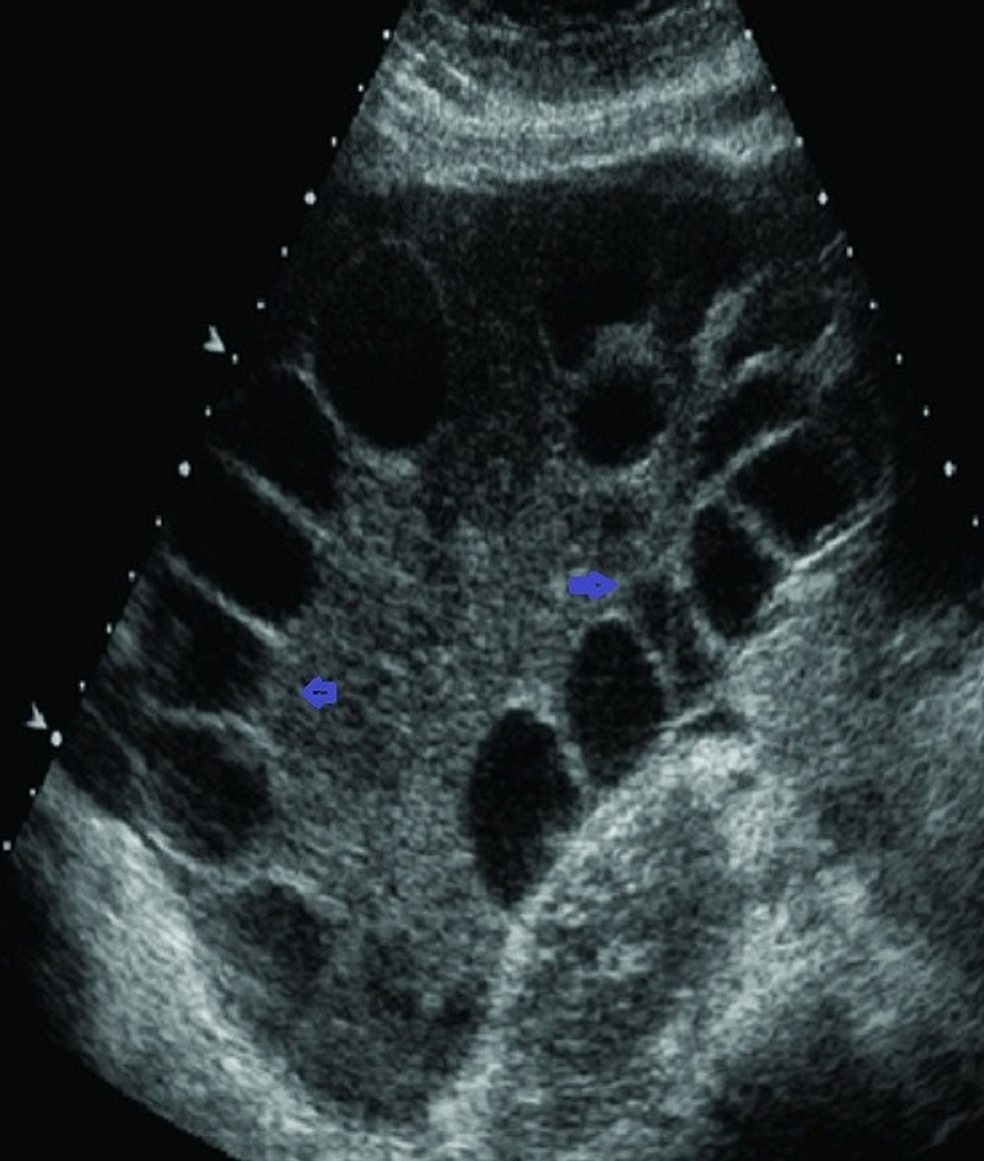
Multiple follicular cysts appreciated on the abdominal ultrasound
